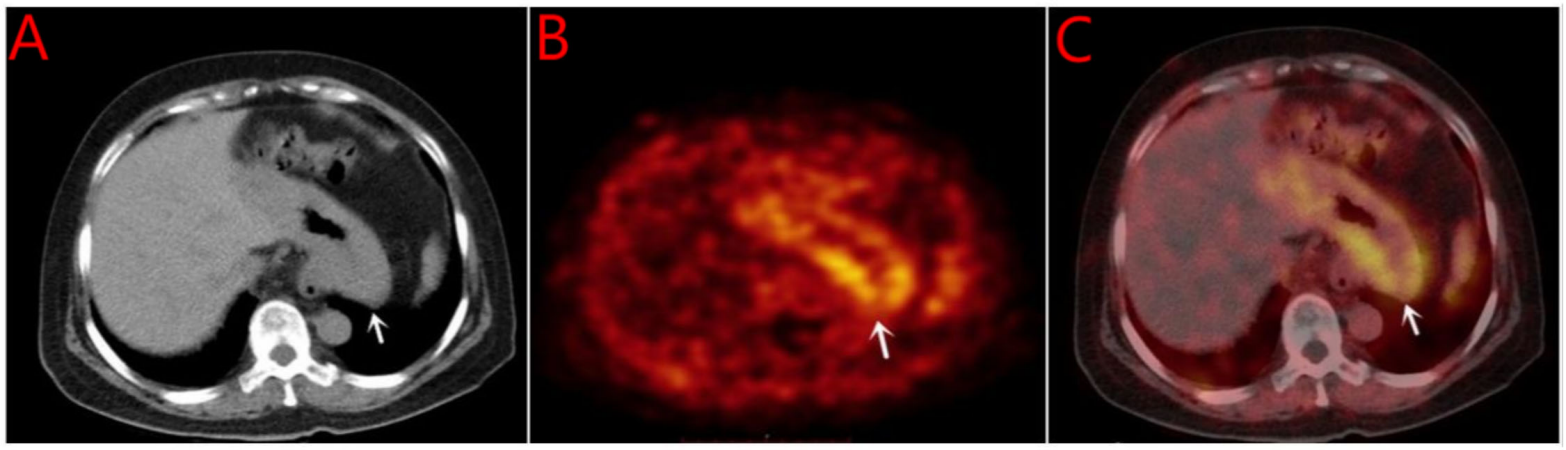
Ga68-FAPI PET/CT before gastric treatment. (A) CT shows thickening of gastric wall; (B) PET shows high metabolism; (C) MIP shows of gastric wall. Ga68-FAPI PET/CT, G68 positron emission tomography-computed tomography; MIP, Maximum Intensity Projection.
